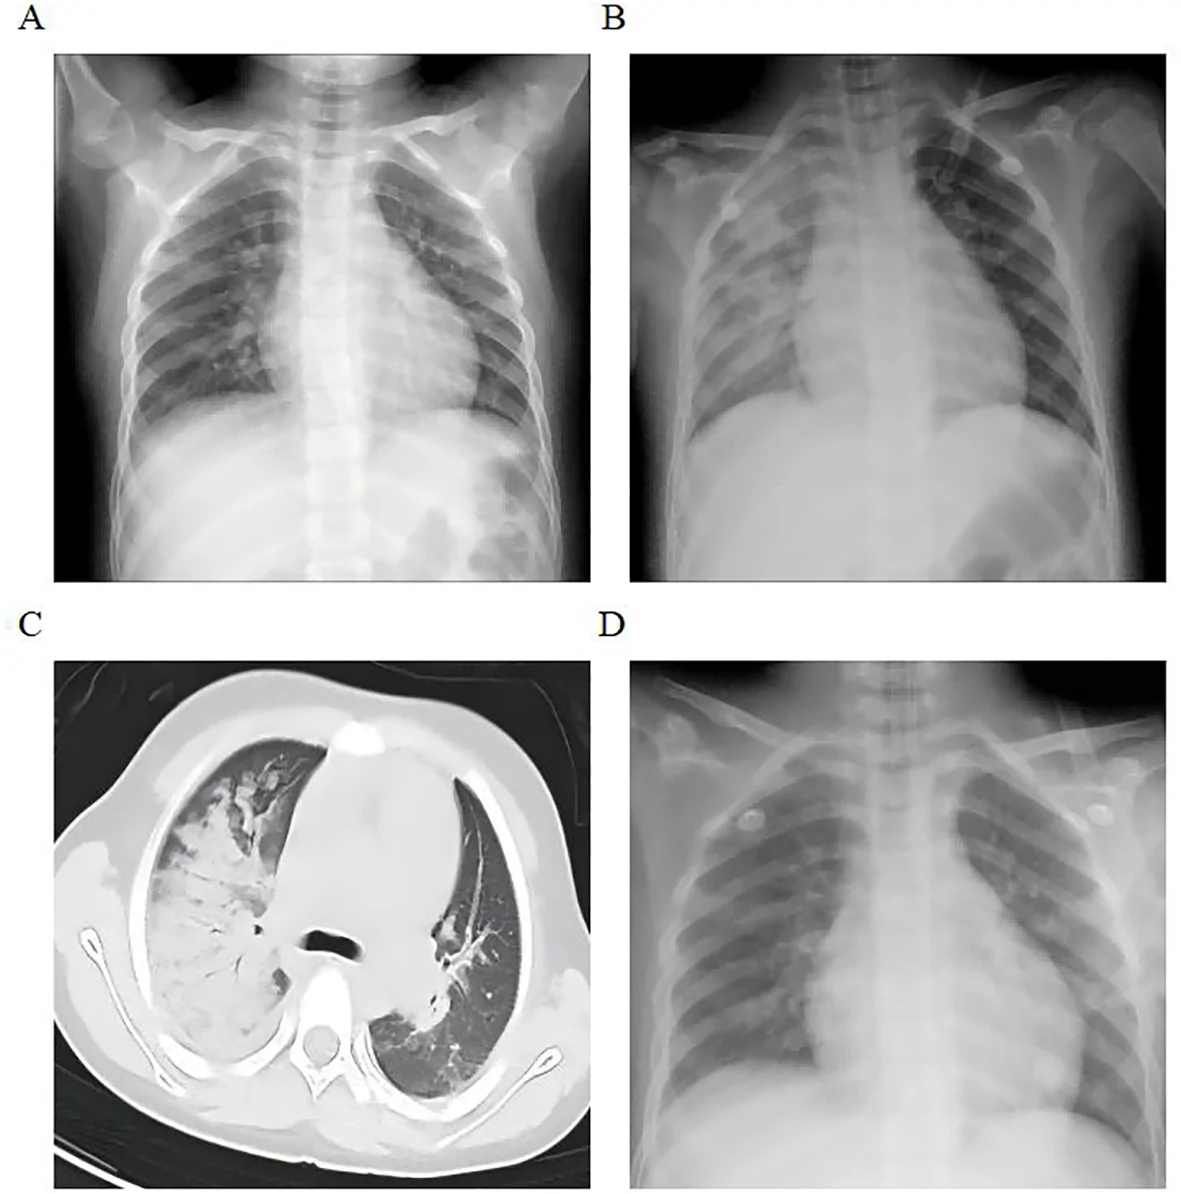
(A) The chest X-ray on admission. (B, C) The chest X-ray and CT on the day of applying invasive mechanical ventilation. (D) The chest X-ray on the day of removing invasive mechanical ventilation.
